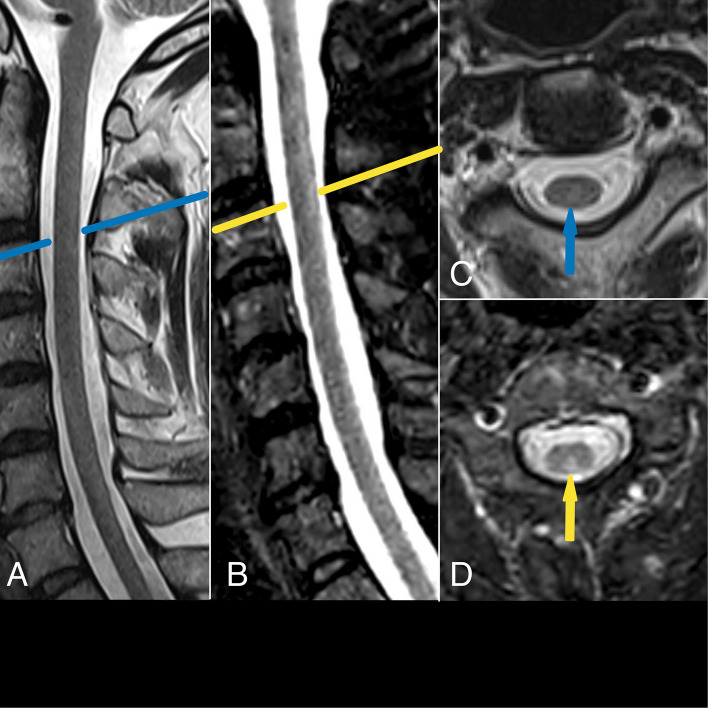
Spinal cord MRI of the patient with T2w-TSE (turbo spin echo) sequences with no apparent signal changes in (A) sagittal orientation and only questionable minimal signal changes in axial orientation (C, reconstruction plane indicated by blue line in A), rated as negative. In a repeated MRI, T2w-STIR sequences were added in sagittal (B) and axial orientation (D, reconstruction plane indicated by yellow line) with clear signal increase in the dorsal columns (yellow arrow) indicating myelopathy
